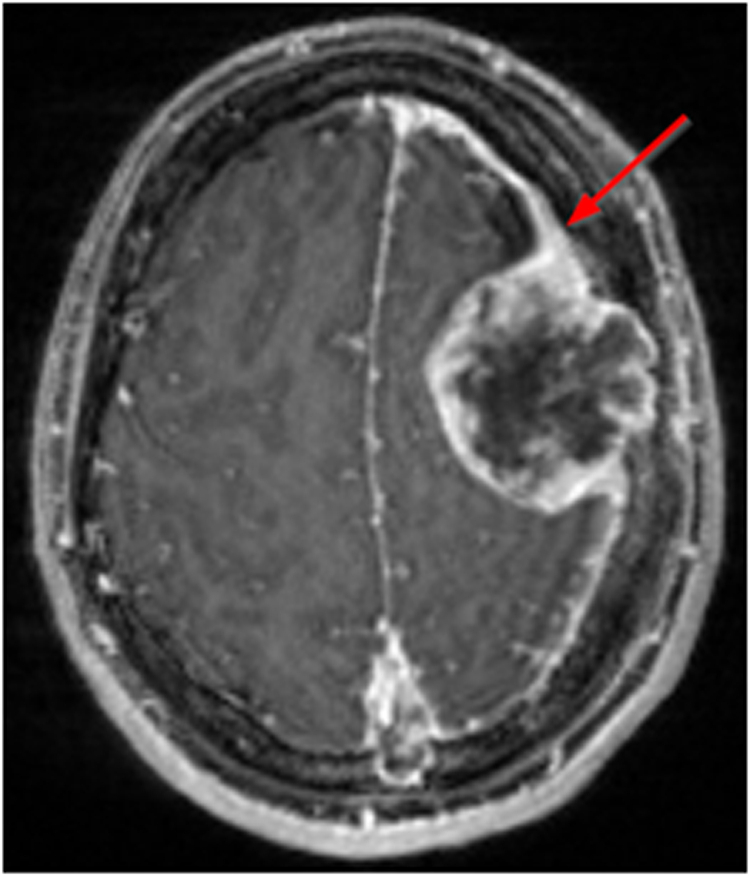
Example of an intracranial meningioma with a dural tail (red arrow) as shown on T1-weighted post-contrast MRI.
